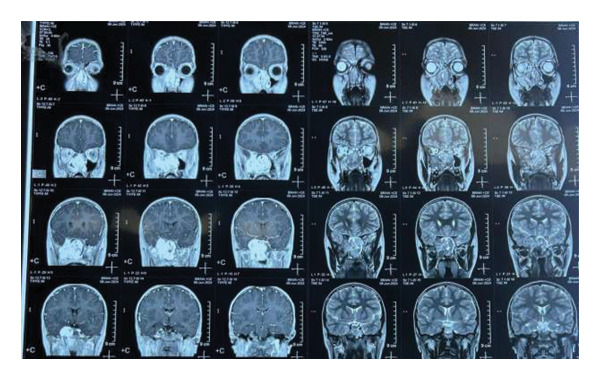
MRI brain with contrast coronal view highlights the mass lesion causing widening of the right pterygopalatine fossa. The lesion extends laterally into the infratemporal fossa, involving the pterygoid muscles, and medially into the posterior part of the left nasal cavity. The tumor’s avid enhancement suggests a vascular nature, correlating with the presence of prominent flow voids indicative of high vascularity.
